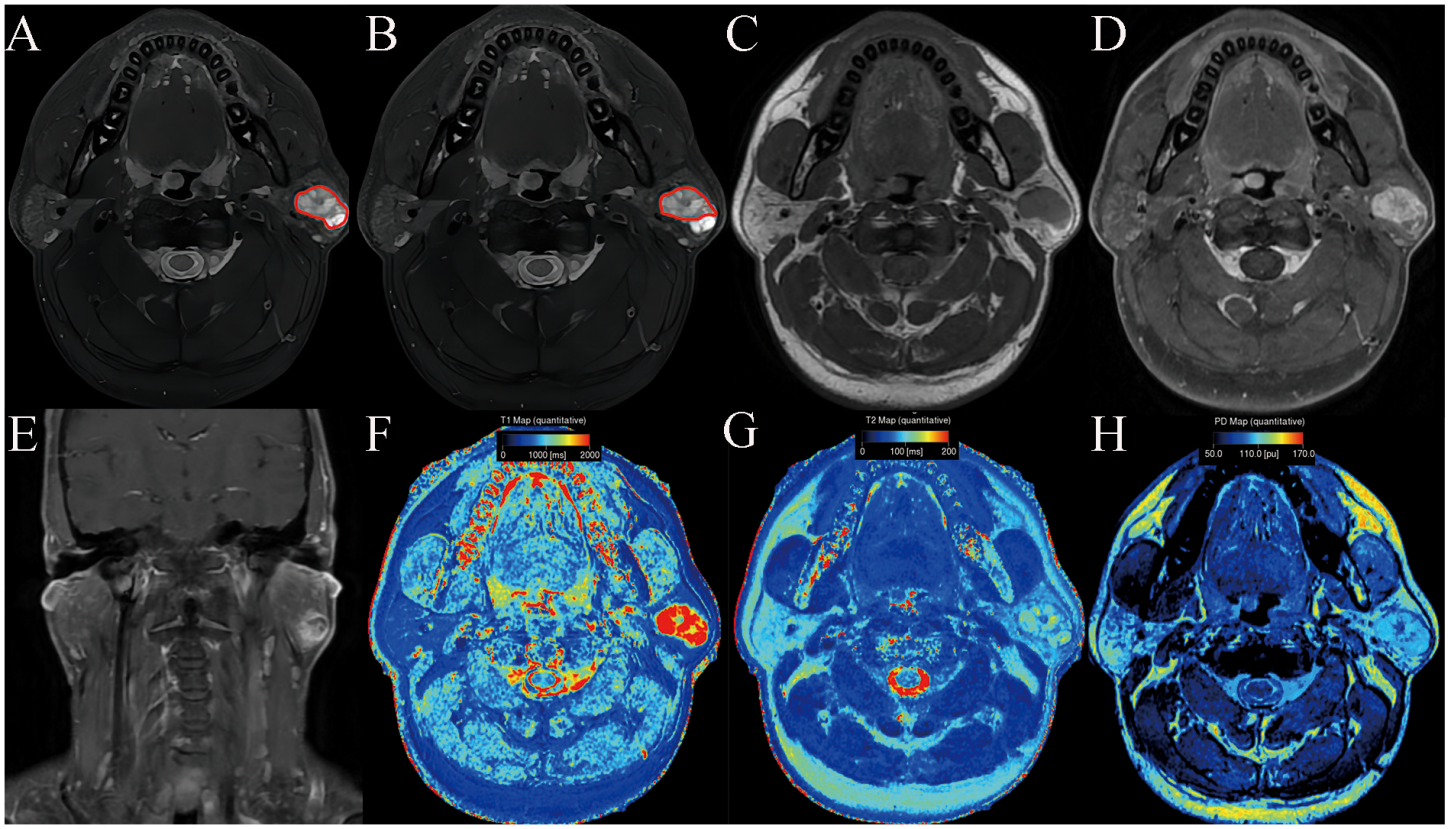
Pleomorphic adenoma of the parotid gland in a 42-year-old male subject. The ROI delineation methods included the largest area of the largest section (full lesion, A) and the solid portion within the largest section (partial lesion, B). On conventional MRI, this mass showed hyperintense on T2WI (A, B), a well-defined border (C), and marked enhancement on contrast enhanced T1WI (D, E). In comparison with WT, it demonstrated significantly higher SyMRI-derived quantitative parameters for partial-lesion ROI (T1: 2077 ms; T1sd: 529 ms; T2: 90 ms; T2sd: 13 ms; PD:99 pu; PDsd: 8.4 pu) on axial T1 mapping (F), T2 mapping (G), and PD mapping (H).
